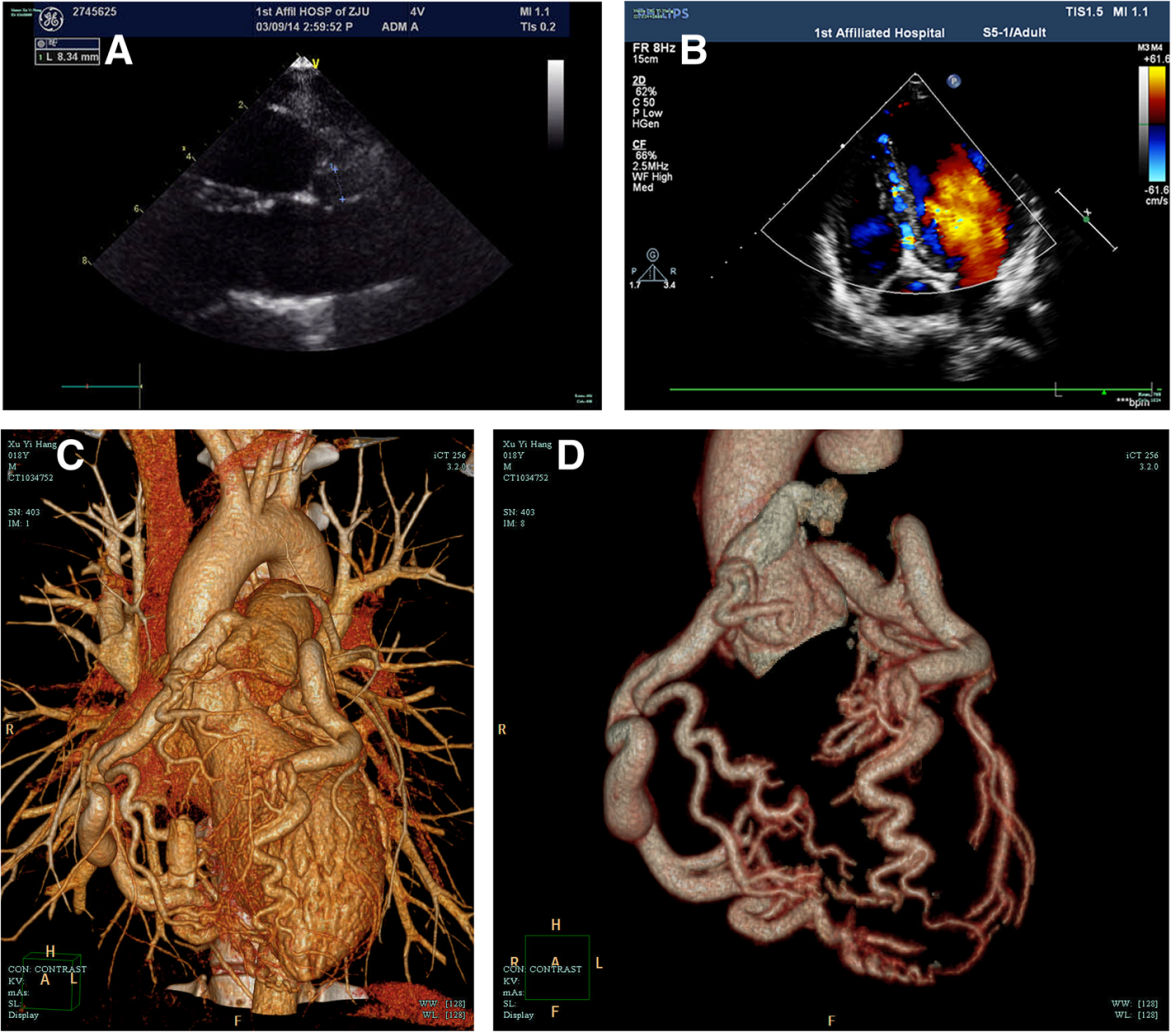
a TTE showed dilated ostium of RCA (white arrows) of about 8.3 mm in diameter; b Doppler echocardiography showed extensive collateralization with continuous flow signals within ventricular septum; c-e Coronary CT angiography showed RCA (white arrows) arose from the pulmonary artery sharing extensive collateralization with LCA (white arrowheads)
